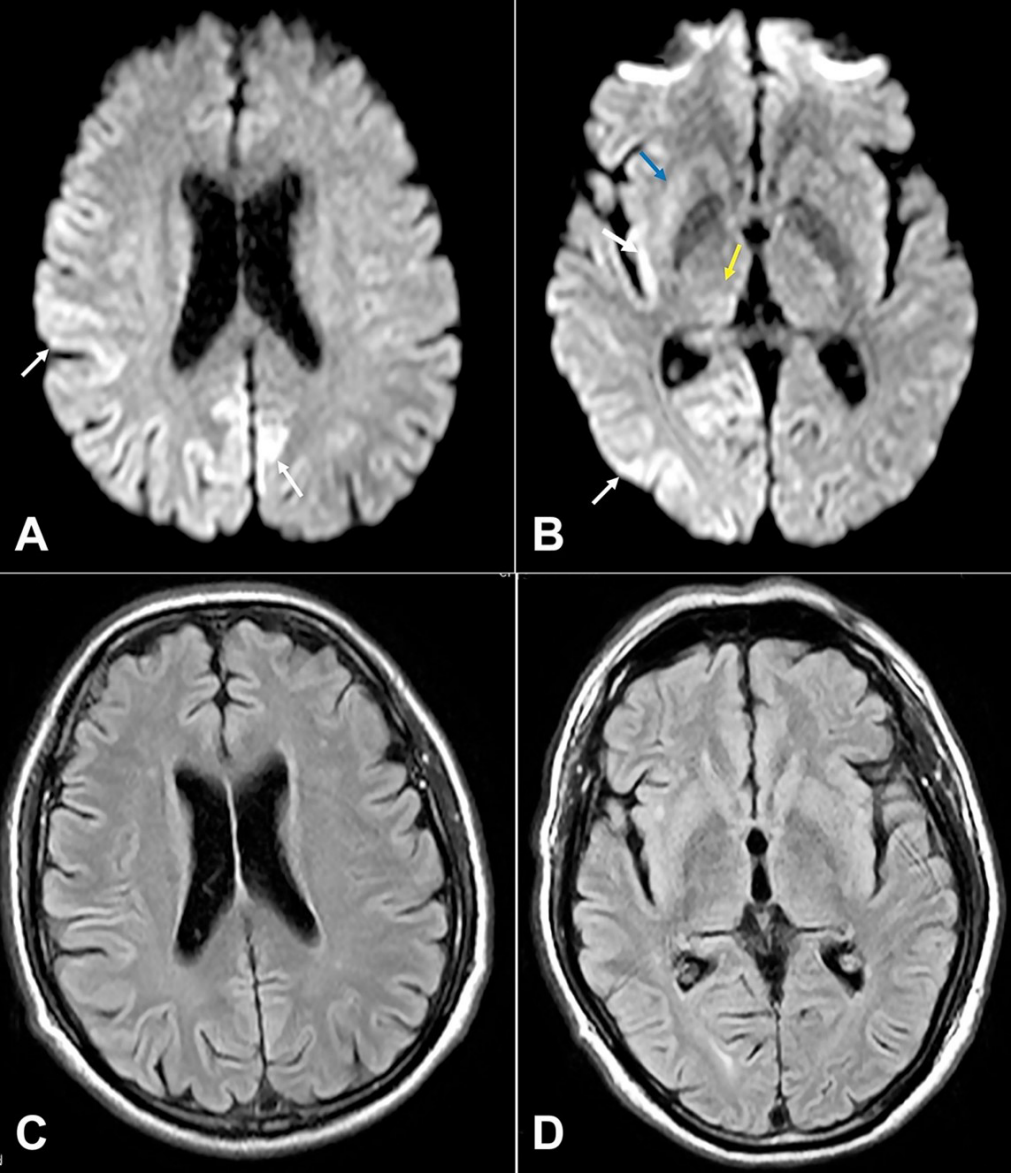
Brain MRI. A – DWI images (b 1000) at basal ganglia; and B – supraganglionic levels showing cortical hyperintensities (white arrows) in the frontal, parietal, occipital lobes and insular cortex, more predominant on the right side (the ‘Cortical Ribbon’ sign - white arrows) and also hyperintensity in the pulvinar of thalami (yellow arrow) bilaterally and the right putamen (blue arrow);C and D – FLAIR images at basal ganglia and supraganglionic levels show subtle hyperintensities in the cerebral cortex in the distribution described above and in bilateral pulvinar region. These findings are consistent with sporadic CJD.
